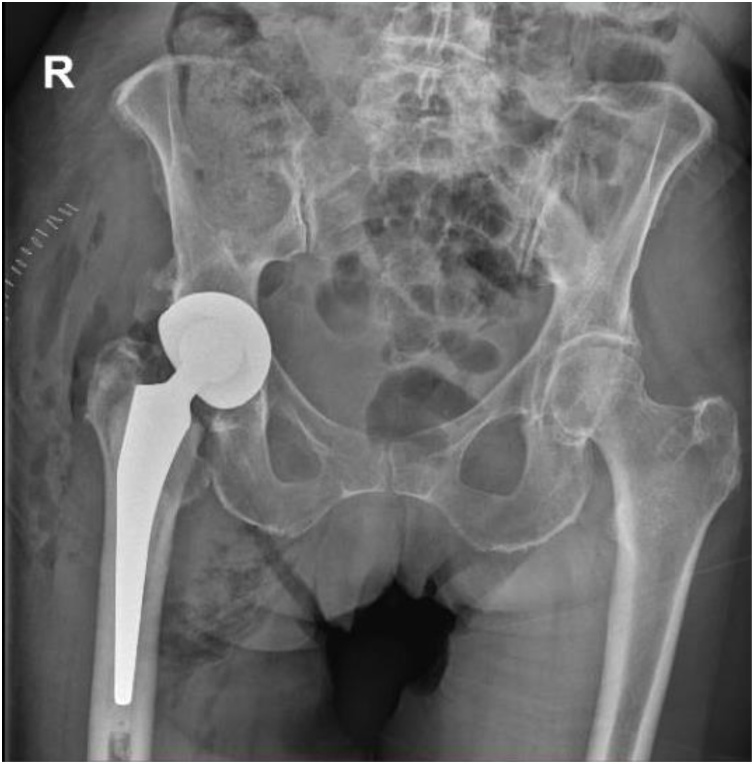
Plain X-ray after revision of the total hip arthroplasty with dual mobility cup and cemented stem.
